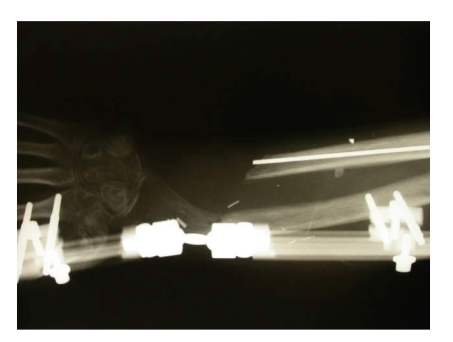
X-ray showing a further displacement of the fracture fragment of the radius, with ulnar deviation of the wrist.
